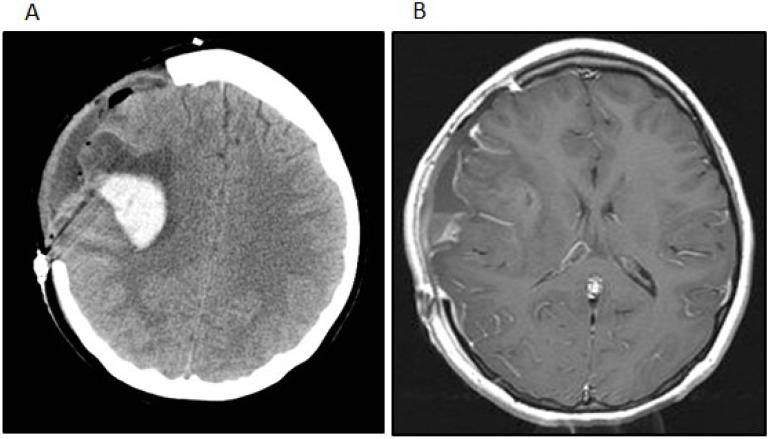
(A) The patient developed a delayed intratumoral hemorrhage requiring hemicraniectomy and evacuation of the hemorrhage and tumor. The noncontrast head CT shows the acute hemorrhage within the right Sylvian fissure extending into the right frontal lobe; (B) The post-contrast t1-weighted image after the second surgery shows subtotal removal of the neoplasm and evacuation of the intratumoral hemorrhage.
